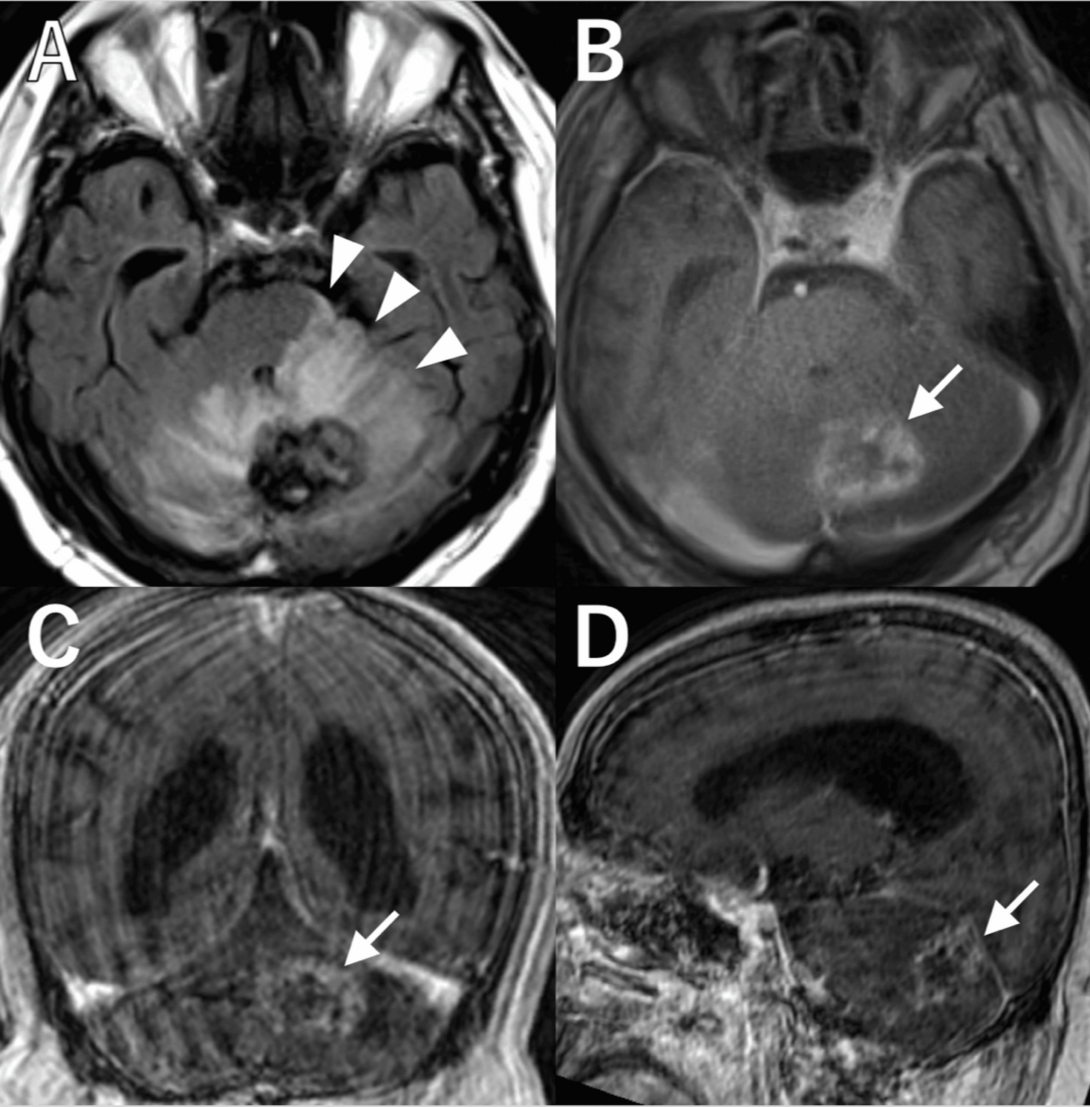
Preoperative images. A-D: Preoperative magnetic resonance imaging demonstrating significant edema in the right cerebellar hemisphere, extending to the contralateral cerebellar hemisphere and brainstem on fluid-attenuated inversion recovery imaging (A, arrowheads) and a ring-enhanced lesion at the prior arteriovenous malformation site on T1-weighted imaging with gadolinium, consistent with radiation necrosis (B: axial, C: coronal, D: sagittal, arrows).
